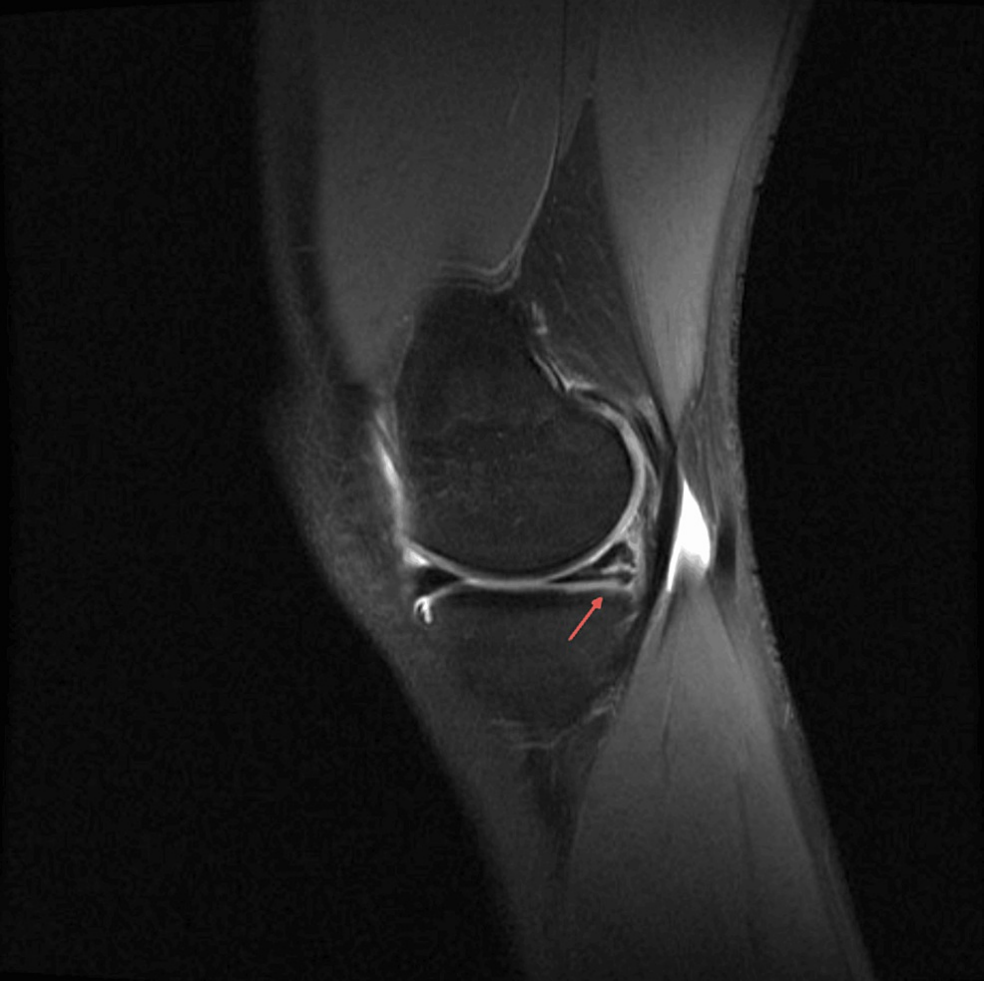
Initial MRI Horizontal tear (red arrow) at the posterior horn of the medial meniscus. Sagittal view of the left knee. MRI: Magnetic resonance imaging
